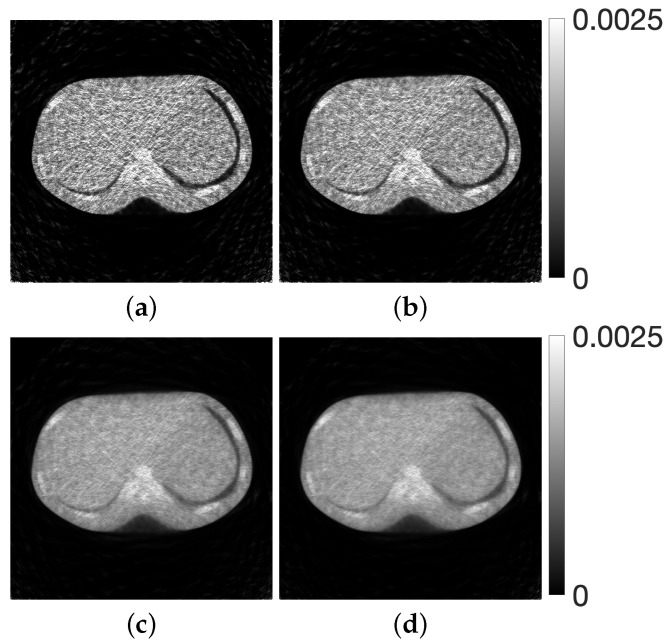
Images reconstructed using CDEM (parameters λ(T)) from projections obtained with an X-ray CT scanner. The distance functions between forward and measured projections are (a) Y2N, (b) YKL, (c) C2N, and (d) CKL.
